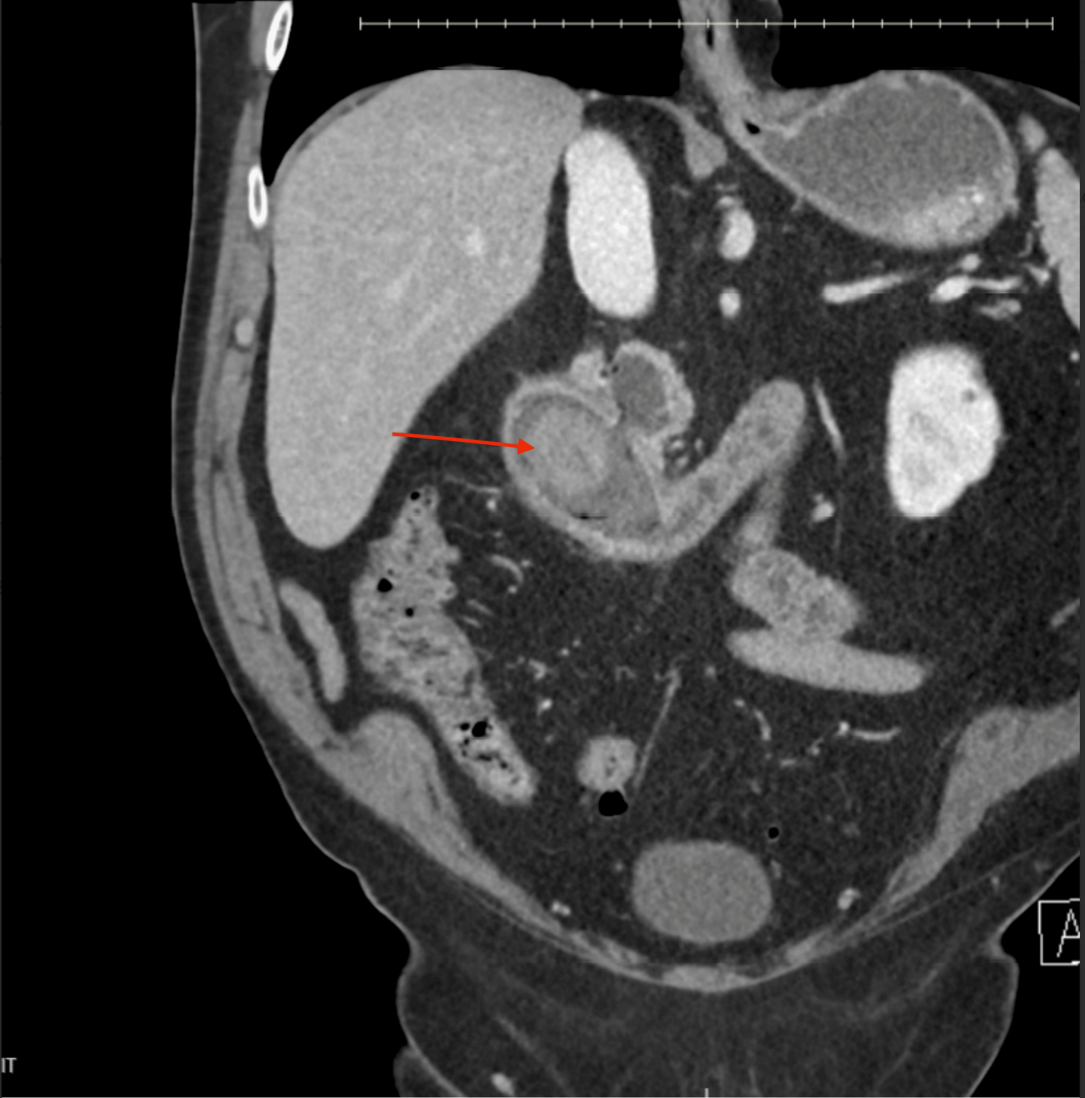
CT abdomen and pelvis demonstrating the large 5.2 x 3.2 cm gallstone (red arrow) within the duodenum along with a cholecystoduodenal fistula, consistent with Bouveret syndrome.
